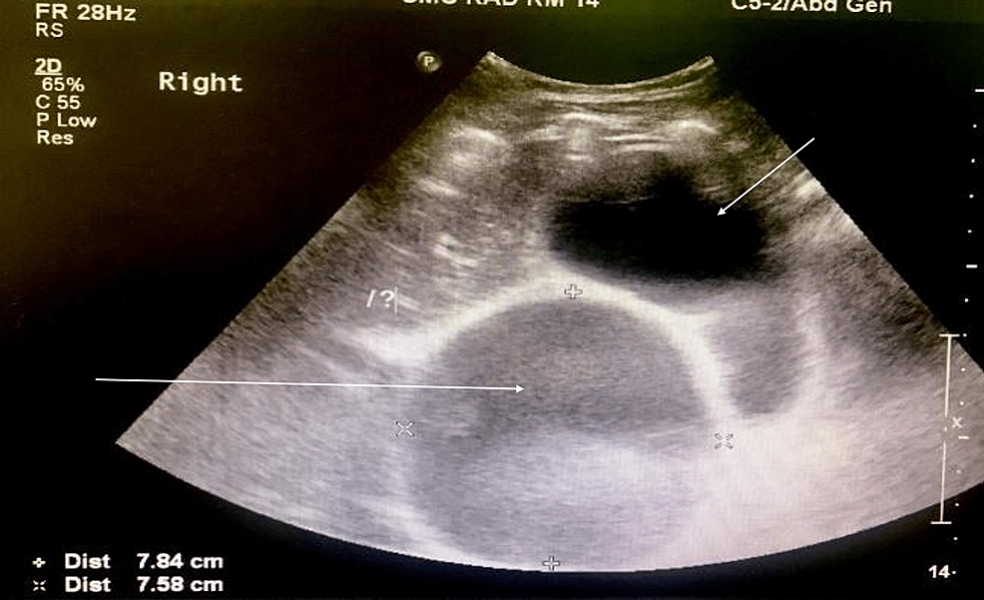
Abdominal ultrasound The short white arrow shows the distended gallbladder, and the long white arrow shows the cyst measuring about 7.8 x 7.6 cm.
